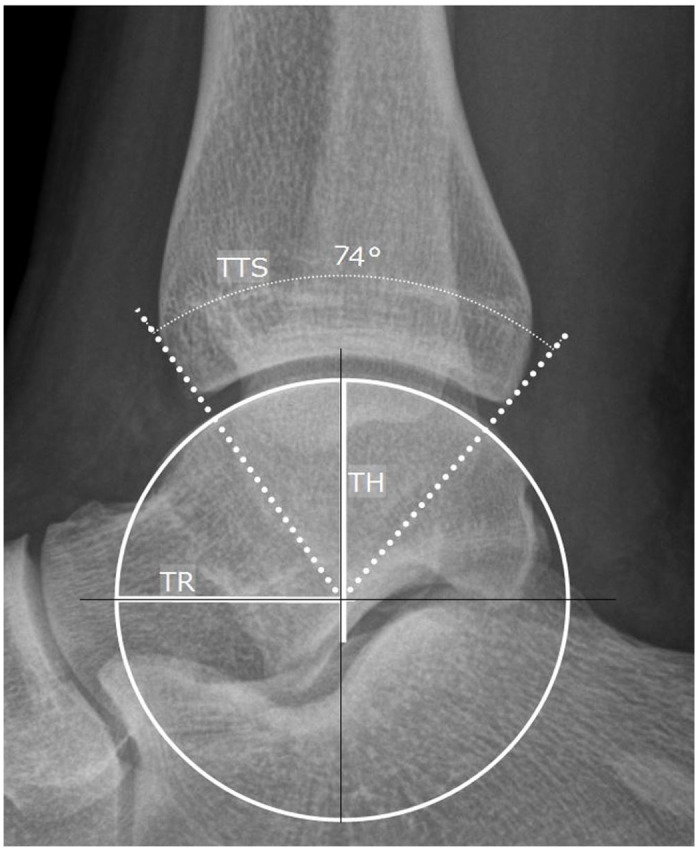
Measurements of the talar radius (TR), talar height (TH), and tibiotalar sector (TTS) on a lateral weightbearing radiograph: the center of the talus was identified by measuring the most appropriate circle on the articular surface of the talar dome. The TR is the straight line from the center of the talus to the circumference of the circle, measured in millimeters. A vertical line to the floor was drawn through the center of the talus. The TH is given by the distance of the vertical line intersecting the articular surface of the talar dome and inferior surface of the talar body, measured in millimeters. From the center of the talus a line was set to the anterior and posterior borders of the distal tibia. The angle formed by these 2 lines represents the TTS, quantifying the extent of tibial coverage over the talus, measured in degrees.
